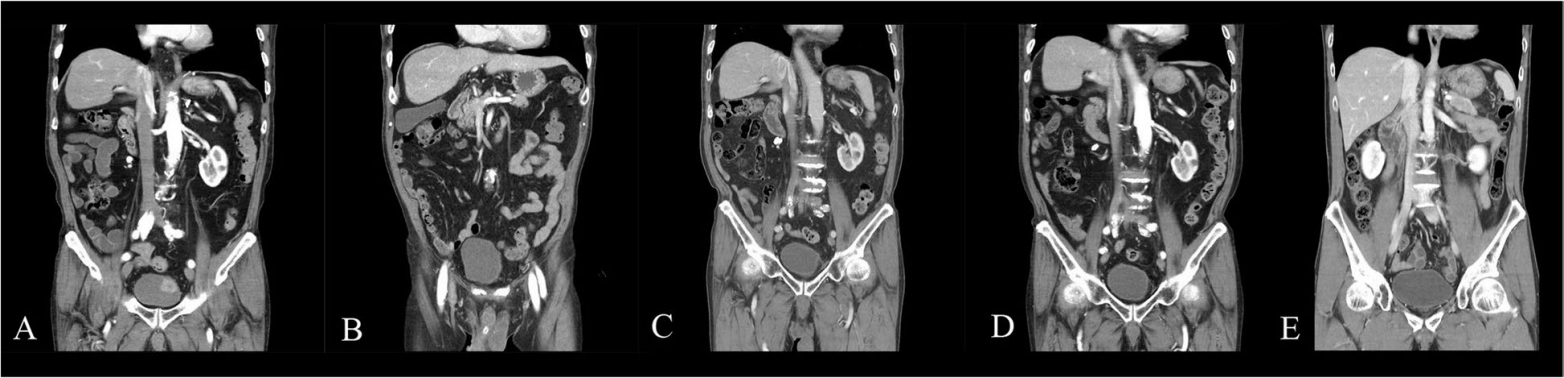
CT scan of partial cystectomy patient. A Preoperative CT scan, B Postoperative 3 months, C Postoperative 6 months, D Postoperative 9 months, E Postoperative 12 months
